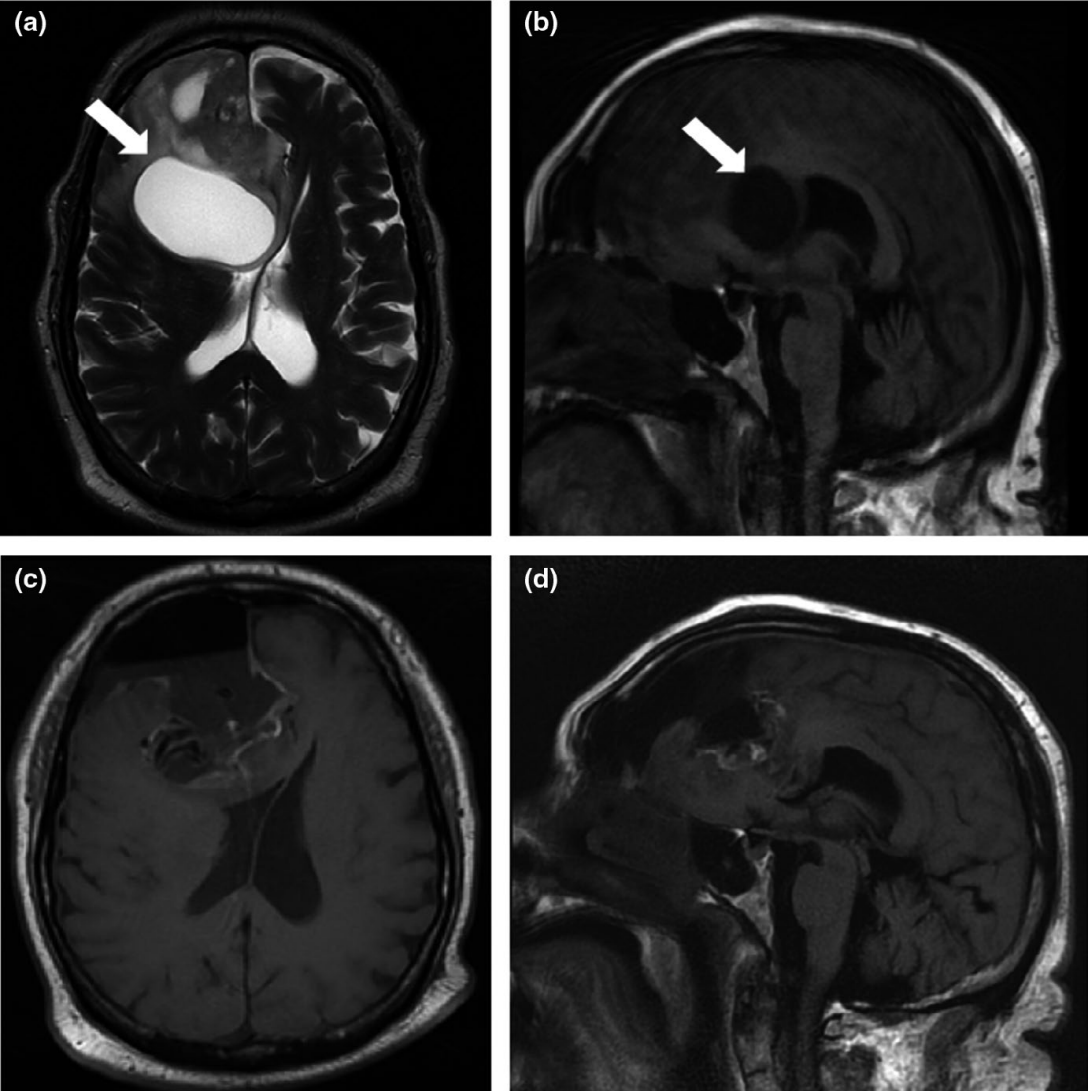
(a) T2‐weighted axial and (b) T1‐weighted sagittal preoperative magnetic resonance imaging demonstrates a large, cystic glioblastoma in the right frontal lobe that extends medially to compress midline structures. White arrows highlight the position of the cystic component of the tumor in panels (a) and (b). (c) T1‐weighted axial and (d) T1‐weighted sagittal imaging demonstrates the extent of tumor resection following awake craniotomy
